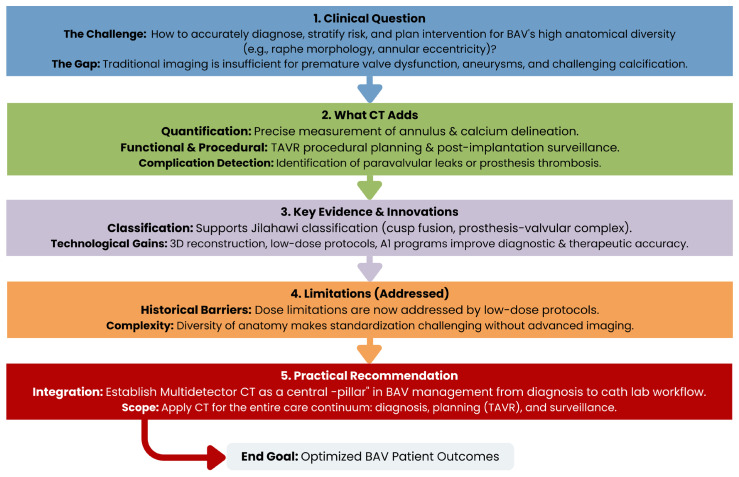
Overview of Cardiovascular CT in Bicuspid aortic valve (BAV) management.
